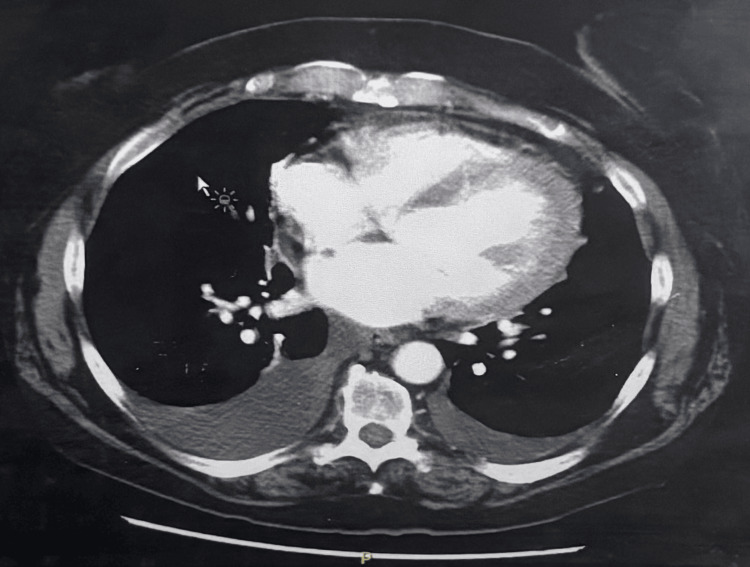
CT scan of the chest showing bilateral pleural effusions, right greater than left, and trace pericardial effusion
